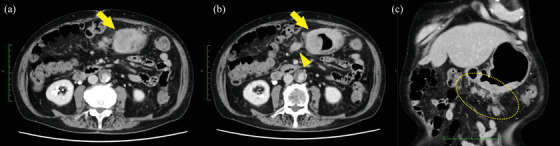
Abdominal computed tomography (CT) and endoscopic findings at diagnosis. Abdominal CT (a–c) shows an unevenly strengthened wall of the lower body of the stomach (arrow), lymph node metastases (arrowhead), and peritoneal dissemination around the stomach (dotted circle).
